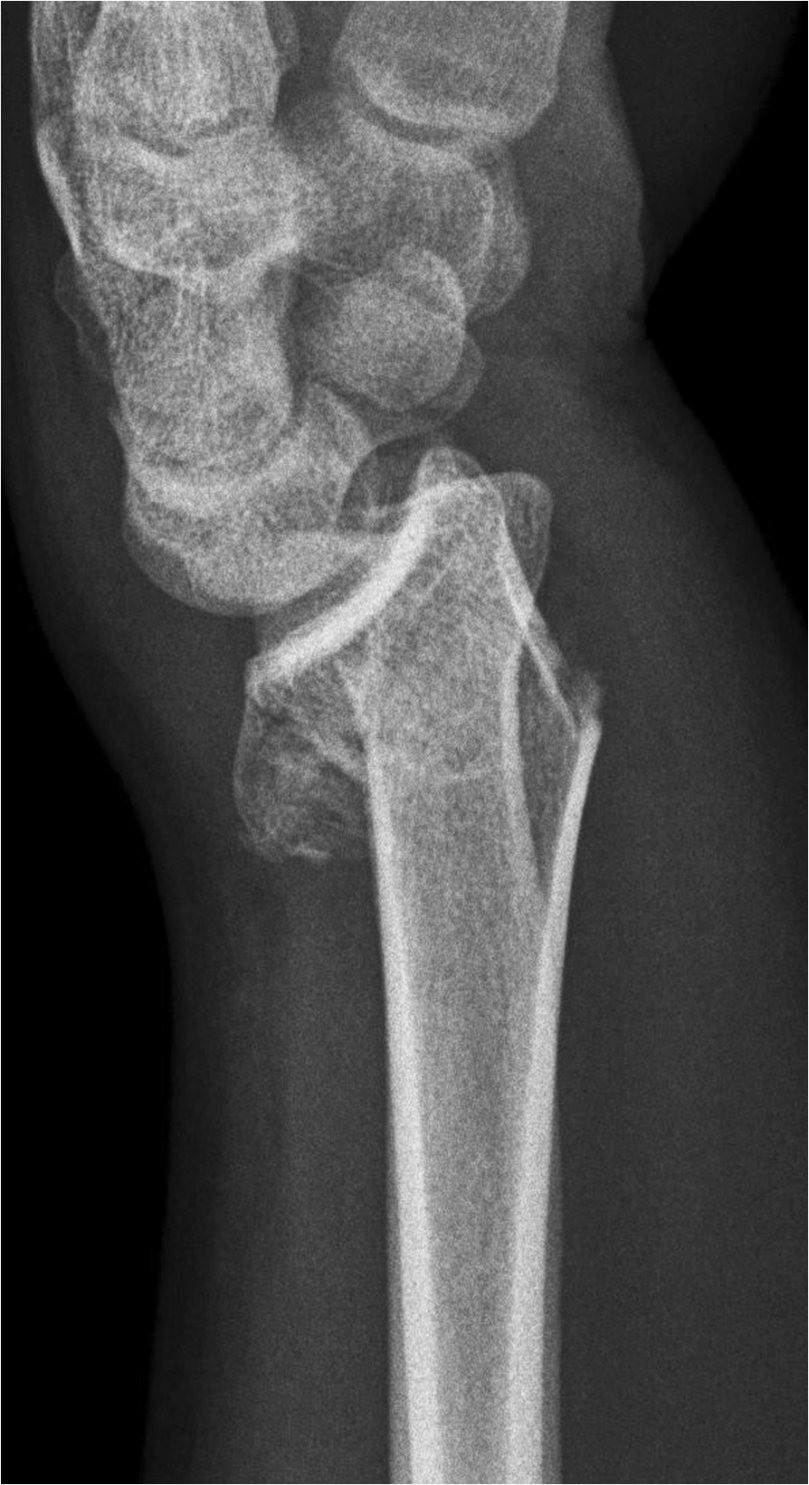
Unstable distal radius fracture; X-ray lateral view.
